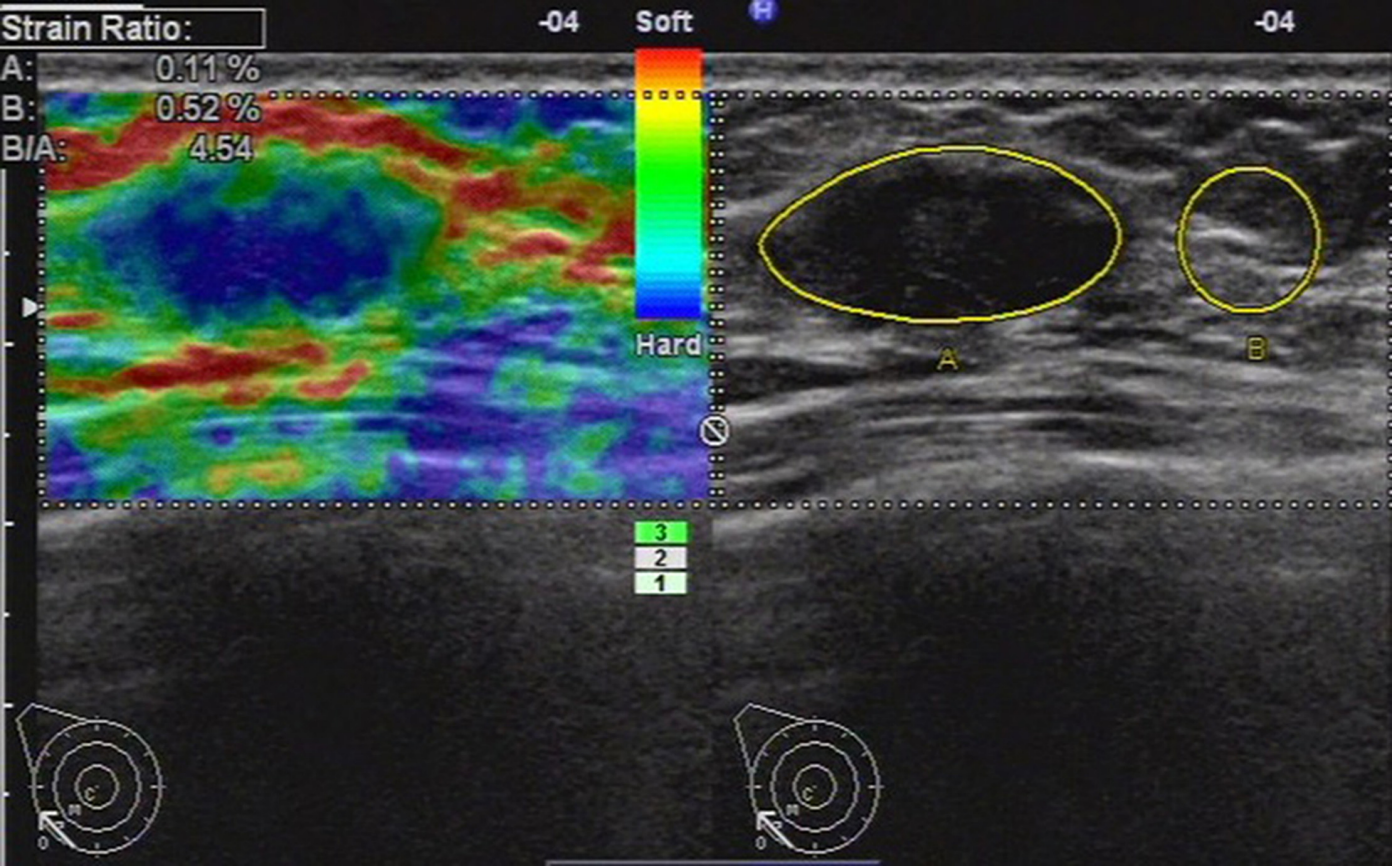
Ultrasonic elastographic image of a papillocarcinoma in a 46-year-old woman. The lesion was scored 4 with the 5-point scoring method. The strain ratio was 4.54 with the strain ratio method at the same breast tissue depth as the reference.
